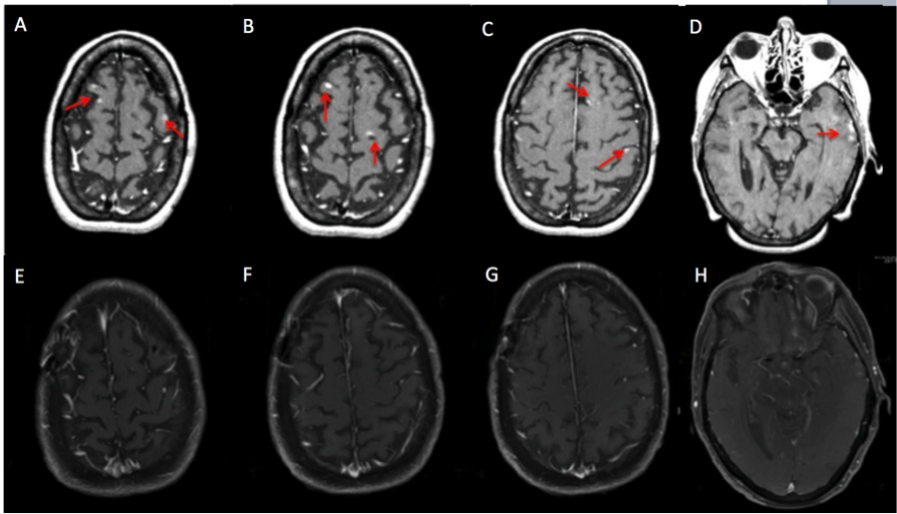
Patient PG presented with 17 lesions at the time of treatment planning. MRI of the brain including A) a right frontal lesion and a left frontal lesion, B) a right anterior frontal lesion and left posterior frontal lesion, C) a left frontal lesion and a left parietal lesion, and D) left temporal cluster. All these lesions were treated with 18 Gy to various isodose lines in one to three shots. On the most recent MRI of the brain on November 2017 (E-H), all treated lesions have disappeared and there were no new lesions concerning for metastatic disease. MRI - magnetic resonance imaging.
